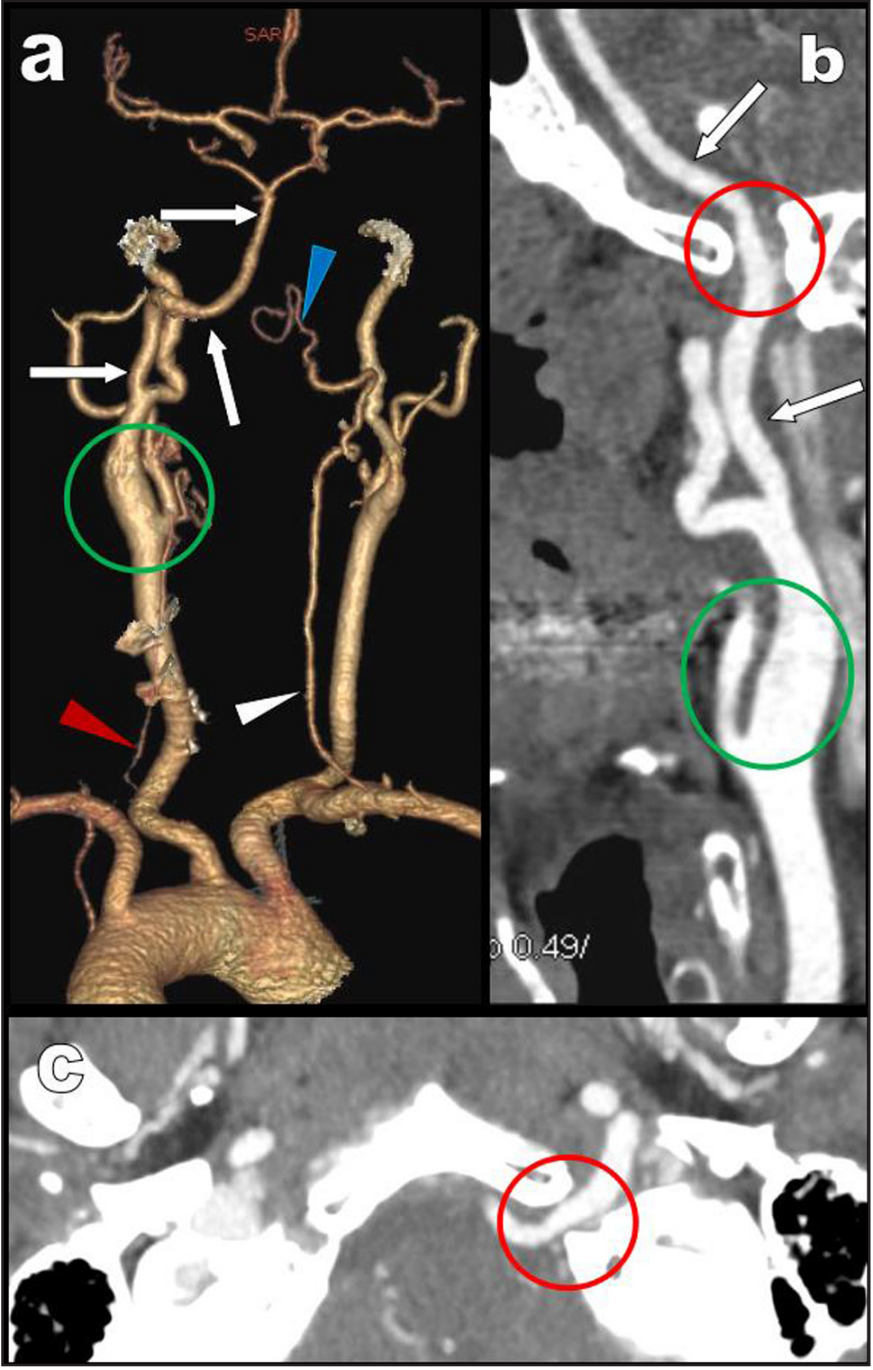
CTA: a) posterior-anterior VR view show major hypoplasia of the vertebral arteries. They are not connected to the basilar trunk which appear only feed by a large ascending artery emerging from a large left internal carotid. Curvilinear reconstruction (b) and axial MIP view (c) at the level of the foramen magna show this large hypoglossal artery penetrating the skull through the supracondylar hypoglossus canal.
